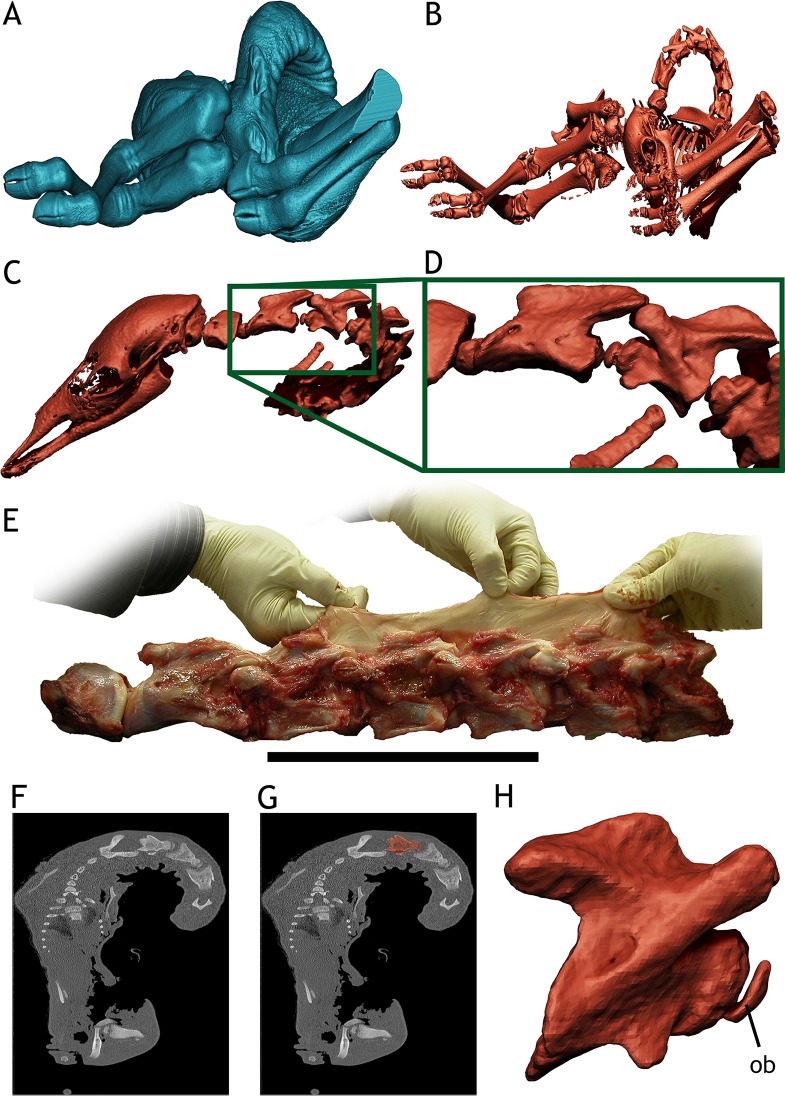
Giraffa camelopardalis* newborn TMM M-16050. A - 3D render of the newborn giraffe body as it was CT-scanned in approximate ventral view. B - 3D render of the newborn giraffe skeleton from CT data, same view as A. C—Cervical vertebrae and skull of the newborn giraffe as it was CT-scanned, showing the intervertebral space. D—Close up on Axis-CV3 of the newborn giraffe, showing the intervertebral space and the ossification body anterior to the condyle. E—Dissected neck from a different newborn giraffe, showing the separation between vertebrae is similar to that of TMM M-16050, modified from Taylor & Wedel [12]. F—CT-scan slide of TMM M-16050. G—Highlight of CV5 on the CT-scan slide from F, showing the ossification body anterior to the vertebral centrum. H - 3D render of CV5 from CT data, showing the ossification body (ob). Scale for E = 200 mm.
